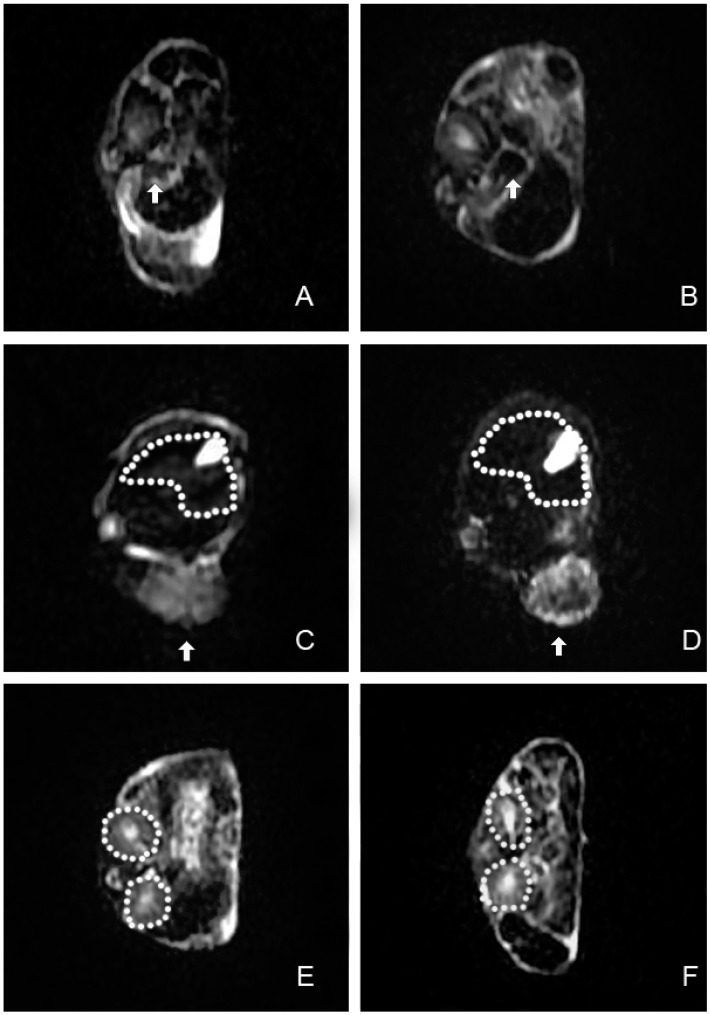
In vivo* MRI images of the spleen, liver, and kidney. (A,B) T2 weighted images of the spleen (arrows) before SPIONs injection and 6 h post-injection; (C,D) T2 weighted images of the liver (dash line) before SPIONs injection and 6 h post-injection. Arrows indicated LNCaP xenograft tumor; (E,F) T2 weighted images of the kidney (dash line) before SPIONs injectionand 6 h post-injection.
